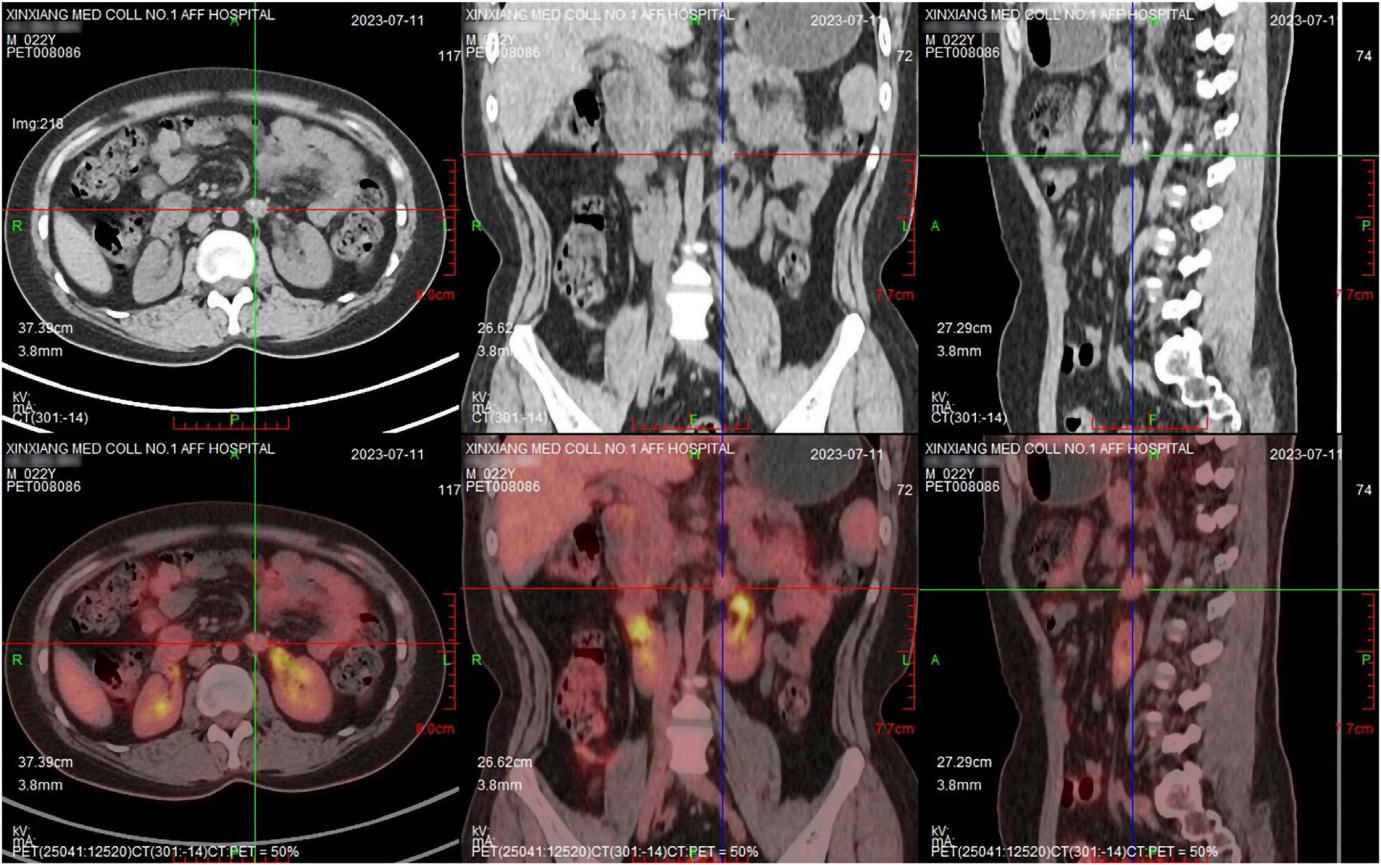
PET-CT after radiotherapy showed the metabolism in the lesion did not increase and enlarged lymph nodes were not found.
